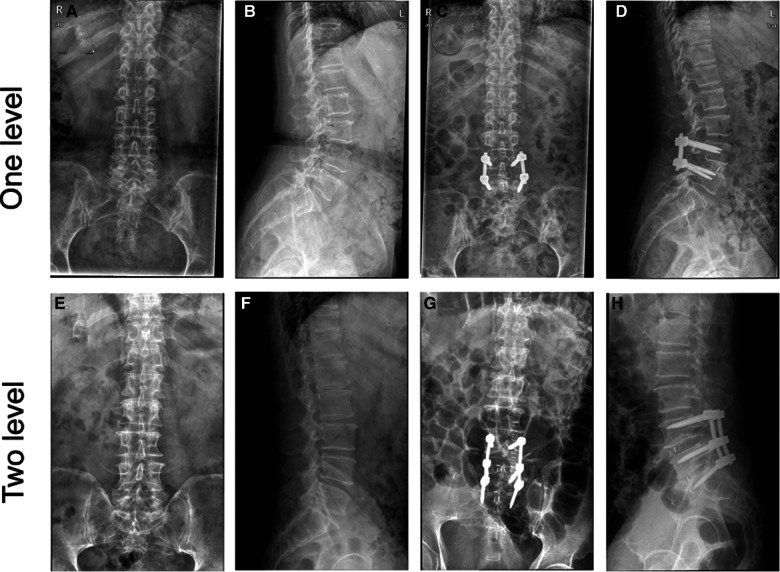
Pre and post-operation 3D TLIF x-rays. (A) Preoperative anteroposterior x-ray of one level TLIF. (B) Preoperative lateral x-ray of one level TLIF. (C) Postperative anteroposterior x-ray of one level TLIF. (D) Postperative lateral x-ray of one level TLIF. (E) Preoperative anteroposterior x-ray of two level TLIF. (F) Preoperative lateral x-ray of two level TLIF. (G) Postperative anteroposterior x-ray of two level TLIF. (H) Postperative lateral x-ray of two level TLIF.
